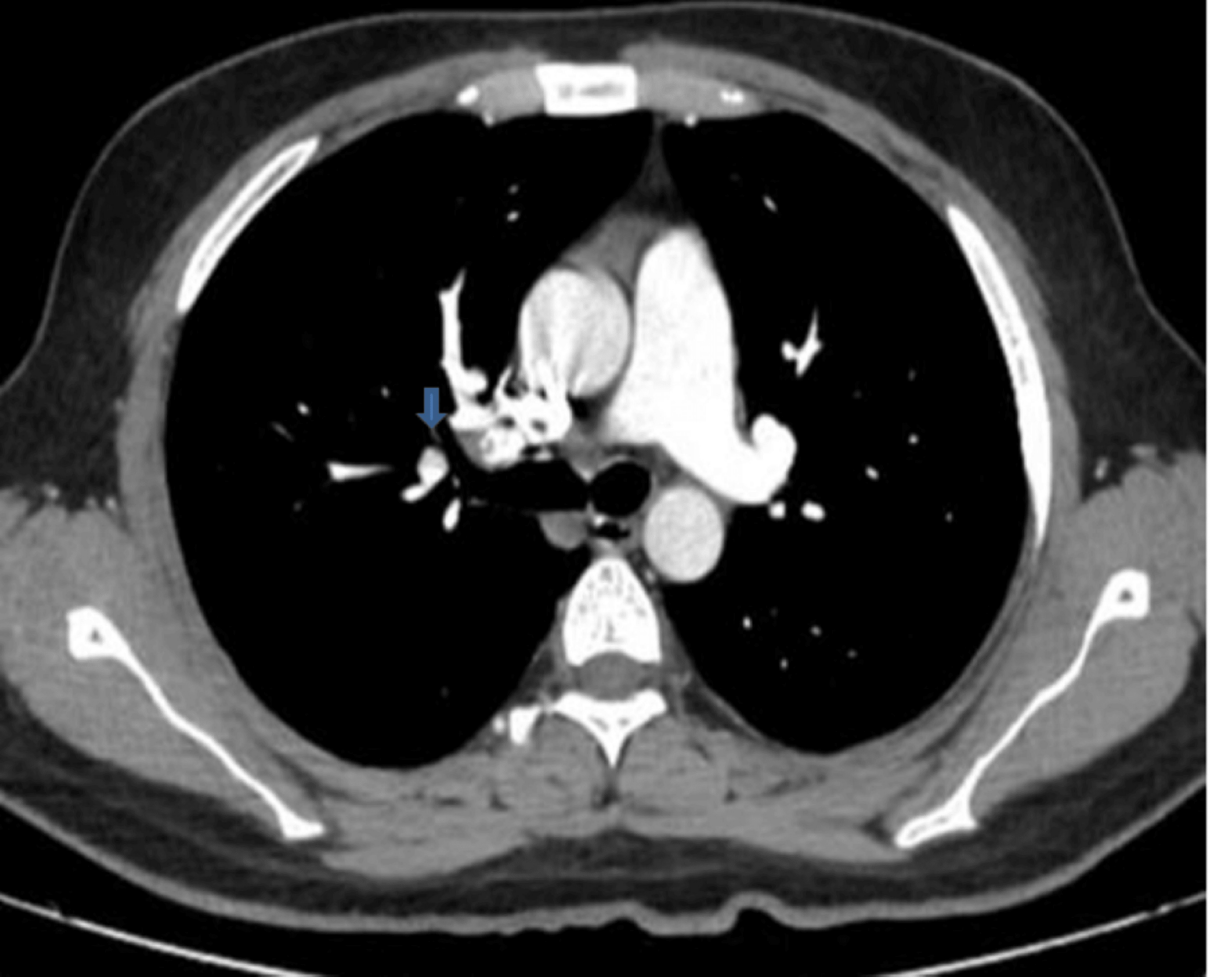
Computed tomography pulmonary angiogram showing thrombus in the right distal pulmonary artery
